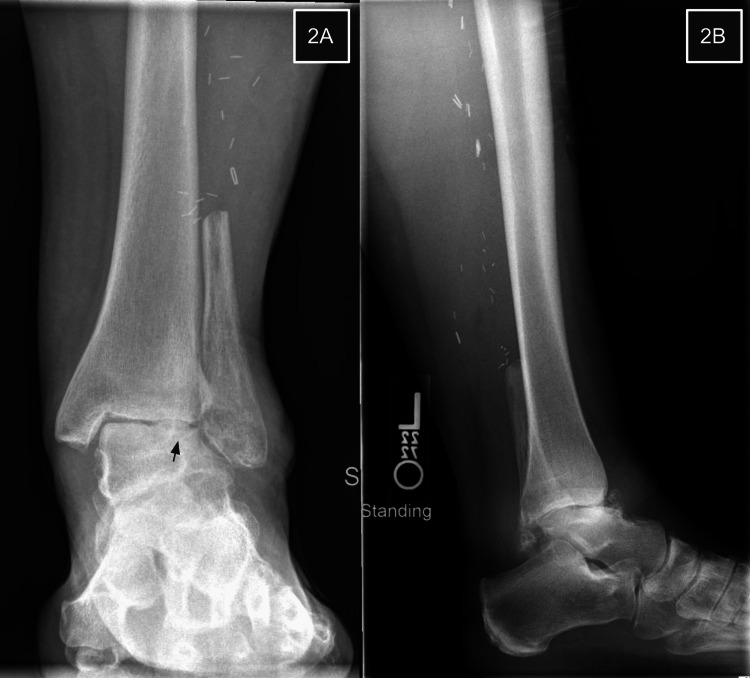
Ankle radiographs nine months post-FVFG 2A demonstrates interval collapse of the talus with valgus tilt and narrowing of the lateral tibiotalar joint and lateral gutter. Proximal displacement of the fibula is noted. The lateral projection (2B) reveals anterior displacement of the talus at the ankle joint.
